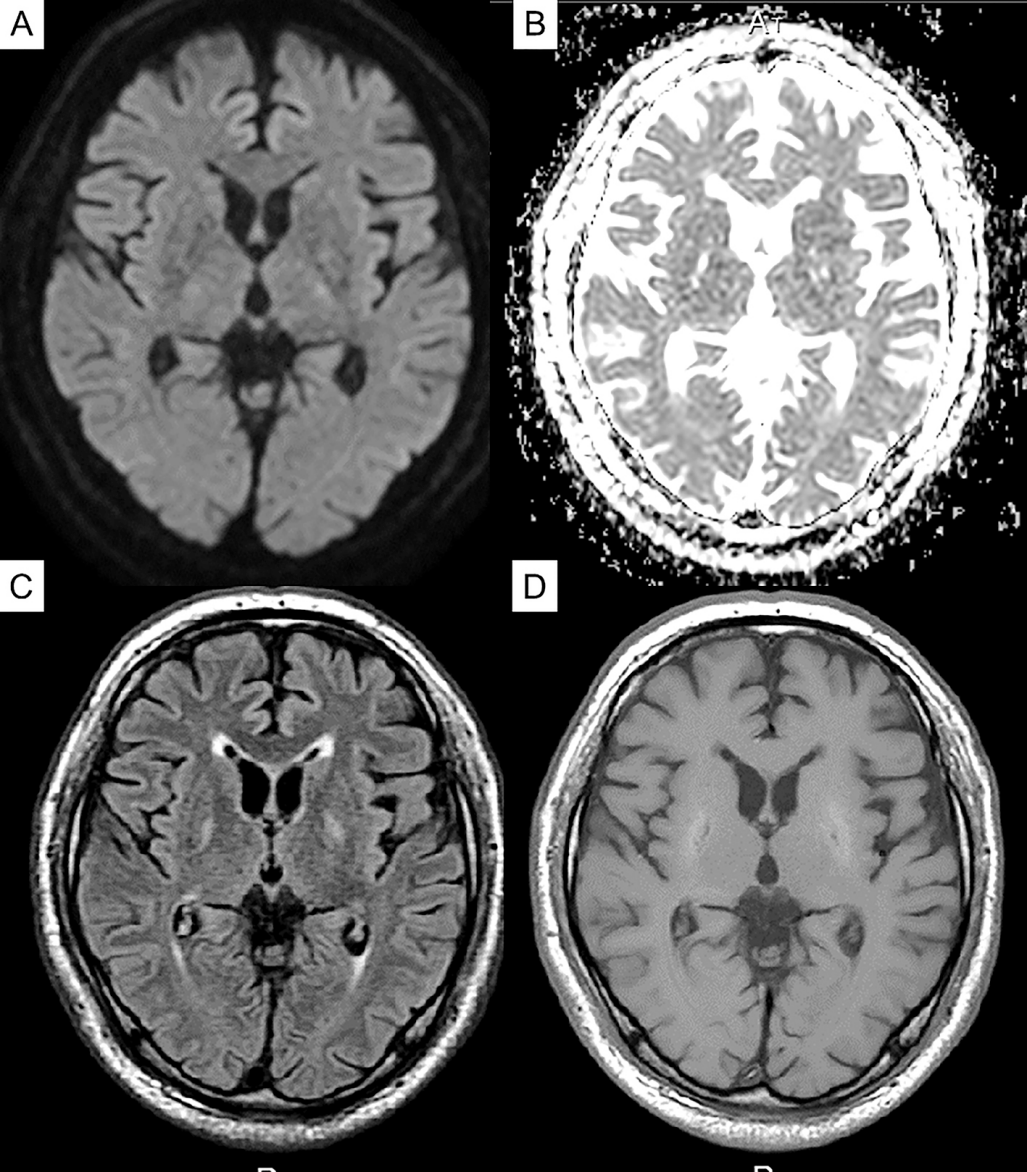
Follow-up magnetic resonance imaging (MRI) obtained on day 30 after admission. (A) Diffusion-weighted image. (B) Apparent diffusion coefficient (ADC) map. (C) Fluid-attenuated inversion recovery (FLAIR) image. (D) T1-weighted image. FLAIR revealing a slightly high signal in both basal ganglia, which were significantly weaker than the initial MRI signal. FLAIR image and ADC map revealing a slit-like high intensity in the globus pallidus; the intensity of these same areas was decreased on T1-weighted images.
